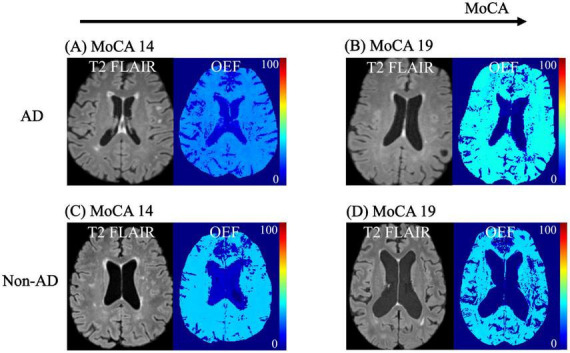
T2 FLAIR and OEF maps in AD and Non-AD dementias. OEF increases significantly with increasing MoCA in AD, from (A) to (B). In the case of Non-AD, OEF does not significantly change as MoCA increases, from (C) to (D).
